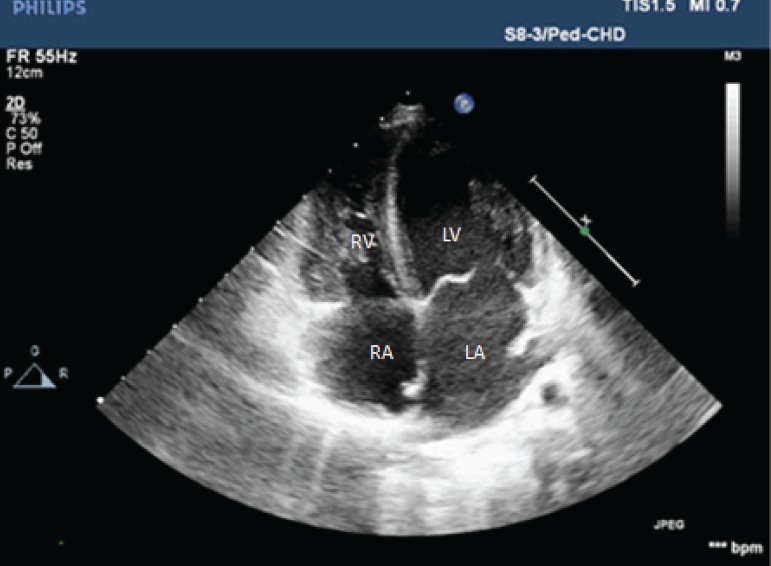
Postoperative 20^th^ day. Apical four-chamber transthoracic echocardiography. LA=left atrium; LV=left ventricle; RA=right atrium; RV=right ventricle
